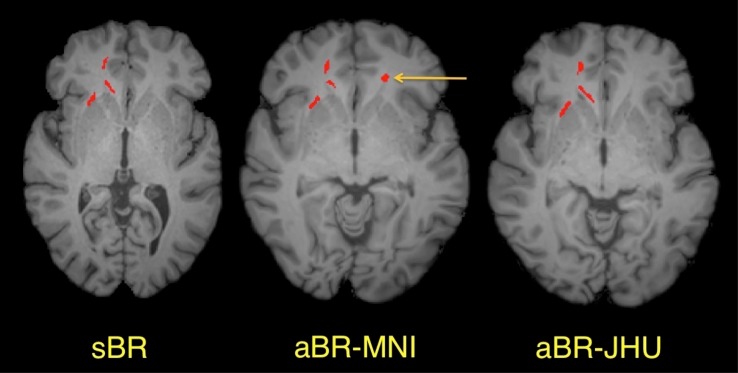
Abnormally low FA clusters detected in a single mTBI patient using three different registration approaches. Abnormally low FA clusters are shown in red overlaid on axial T1-weighted images: the patient’s anatomy (sBR), the MNI atlas (aBR-MNI) and the JHU atlas (aBR-JHU), respectively (left to right). Most abnormally low FA clusters observed using sBR were also present using the aBR methods, as demonstrated by the 3 clusters seen in the right frontal lobe. However, additional clusters (e.g., yellow arrow) detected using a particular aBR template generally will not overlap with the alternate aBR template or sBR.
